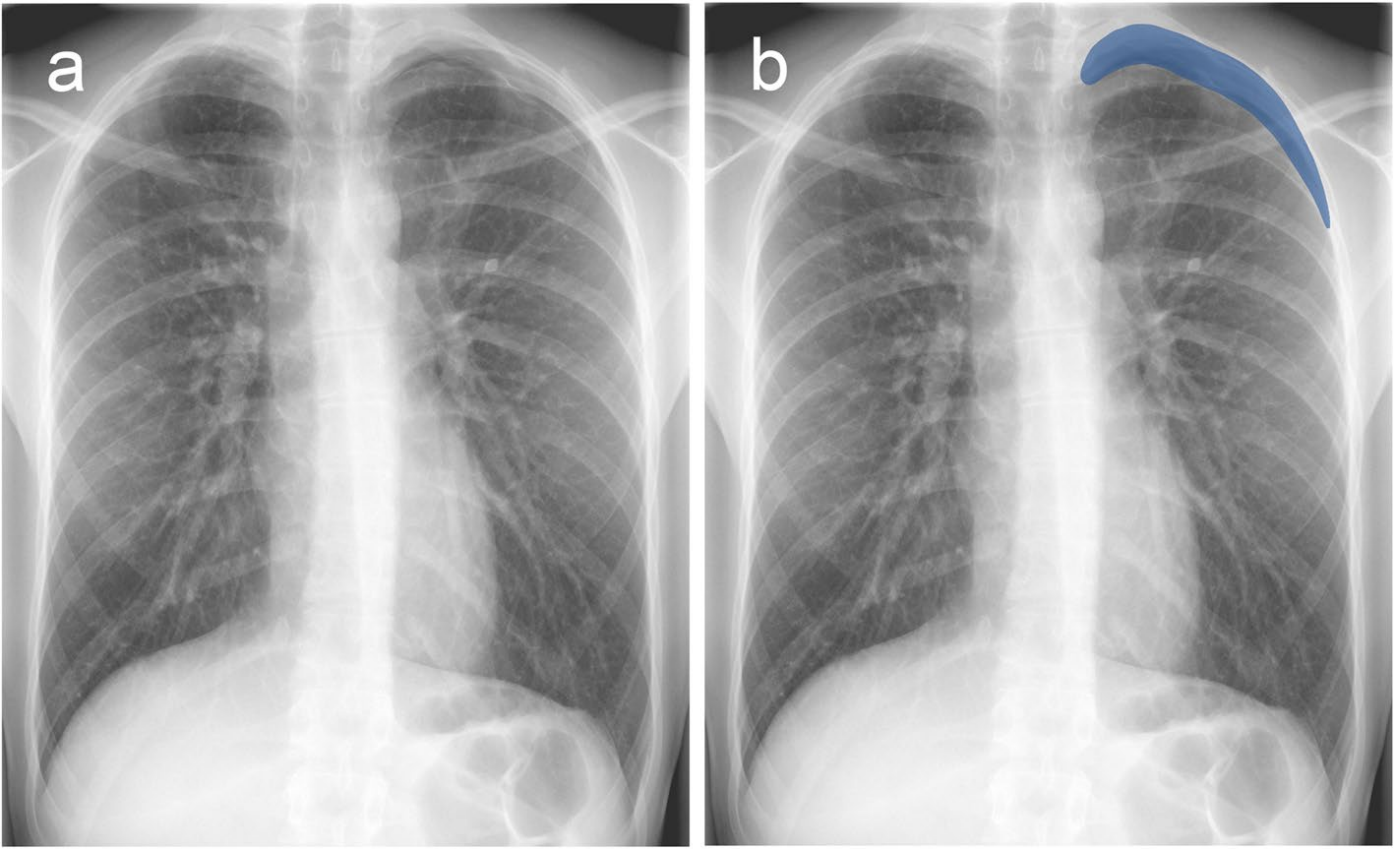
Missed pneumothorax on chest radiograph was detected by AI-assisted double reading. a Frontal chest radiograph of a 20-year-old male patient with chest pain after a previous COVID-19 infection. The radiograph was reported as normal. b The AI software detected a left apical lucency (blue) consistent with a small apical pneumothorax, which was initially missed by the radiologist. AI, artificial intelligence; COVID-19, coronavirus disease 2019
